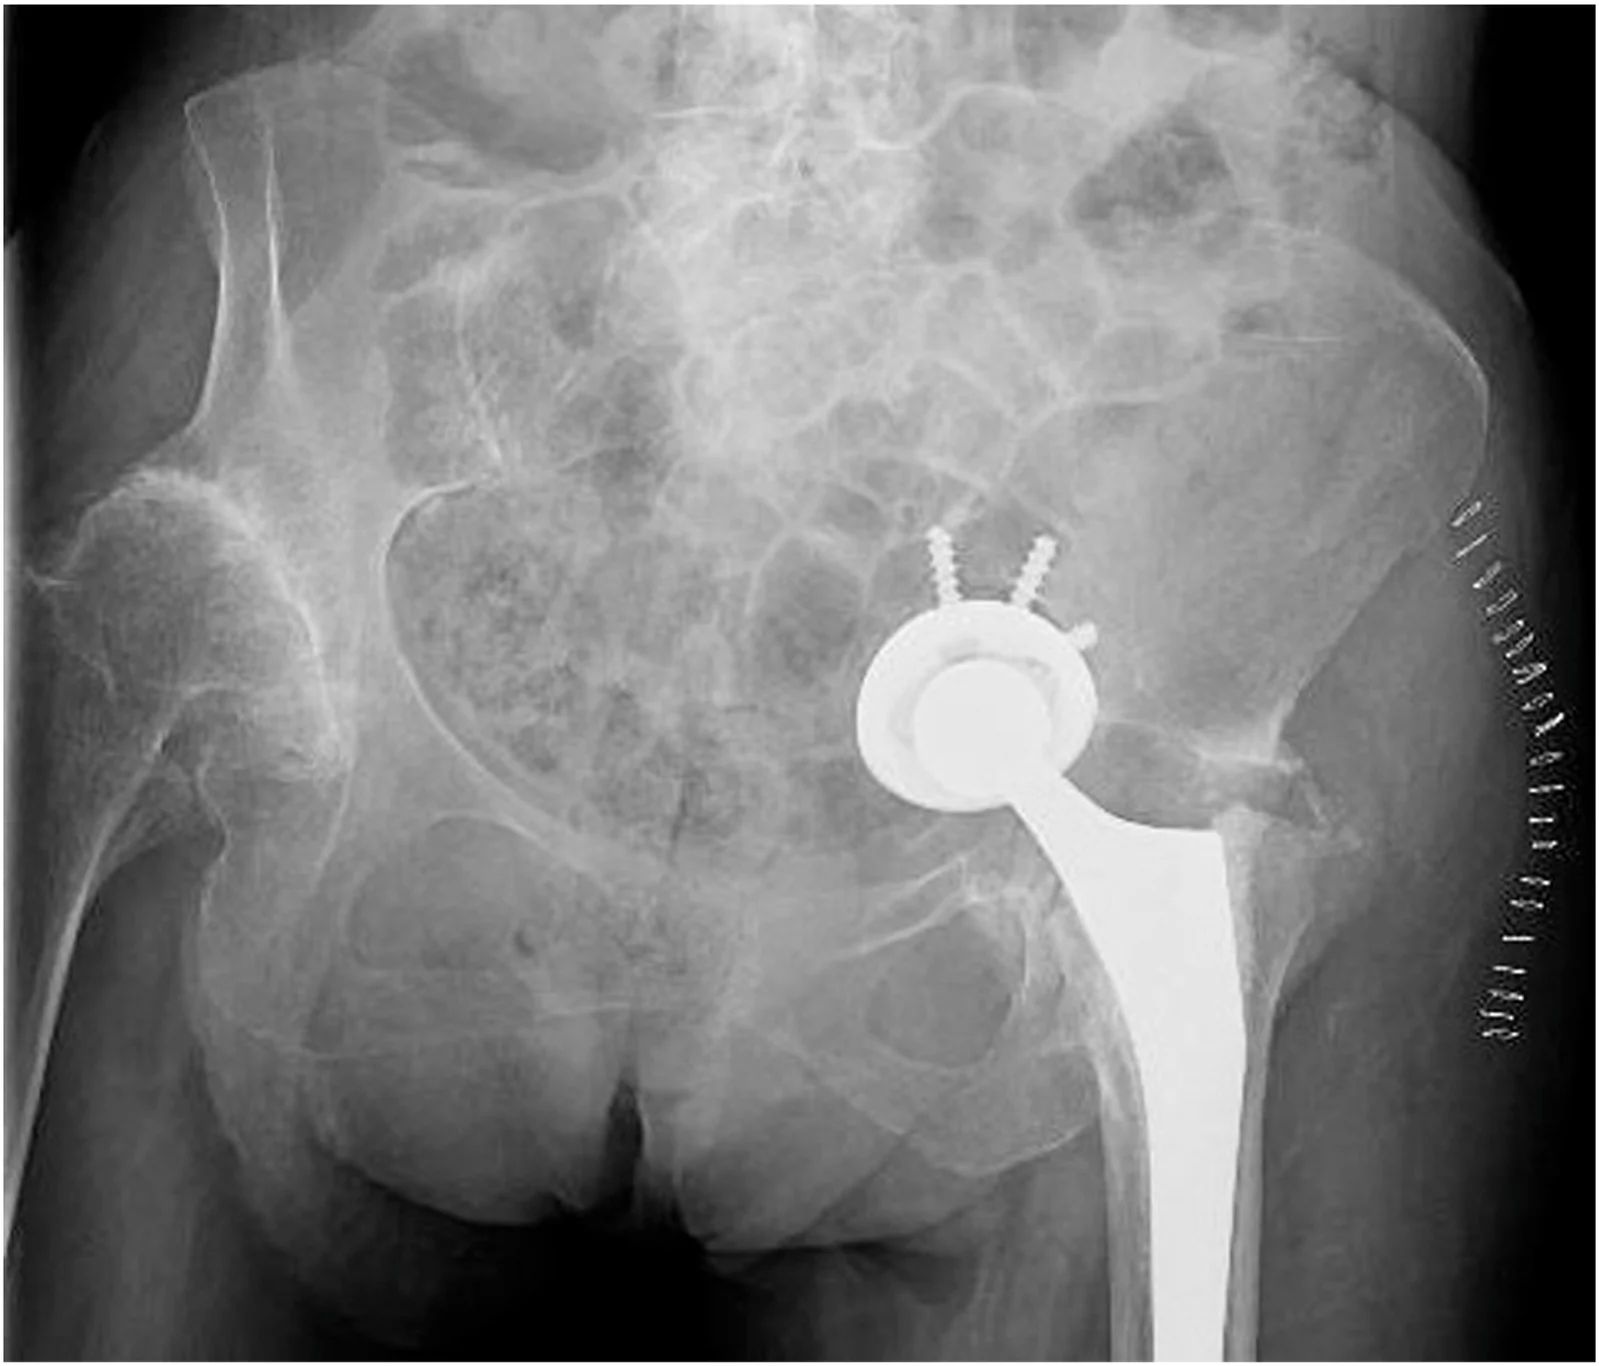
Anteroposterior pelvic radiograph upon presentation (progression of the intrapelvic cup migration).
